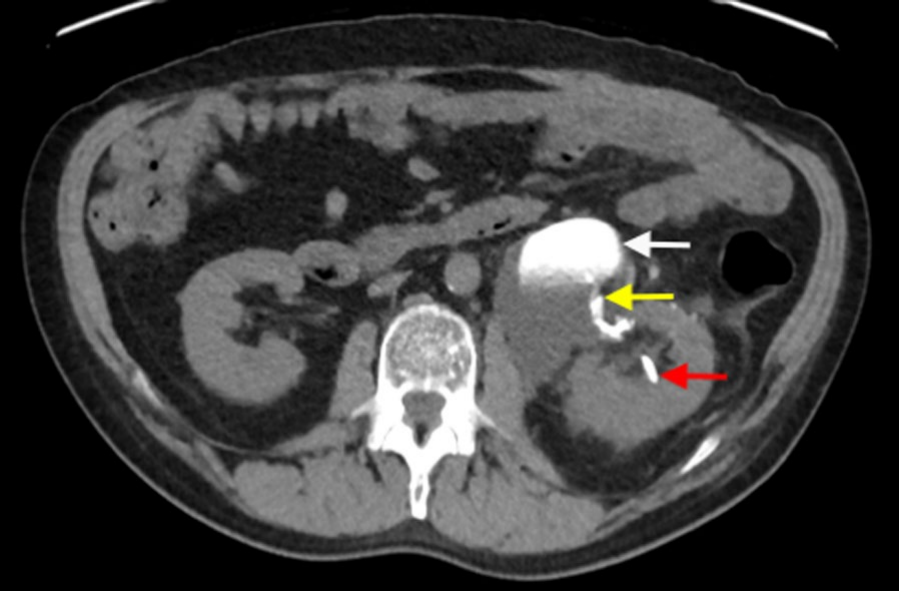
Delayed excretory phase CT image of contrast extravasation into a urinoma (white arrow) following left ureteric injury during a left partial nephrectomy. The yellow arrow demonstrates the site of active contrast extravasation from the left pelvi-ureteric junction, which was injured during the operation. A nephrostomy catheter (red arrow) was sited to decompress the collecting system and to facilitate urinary drainage. The patient developed persistent pelvi-ureteric junction obstruction and required a completion left nephrectomy
